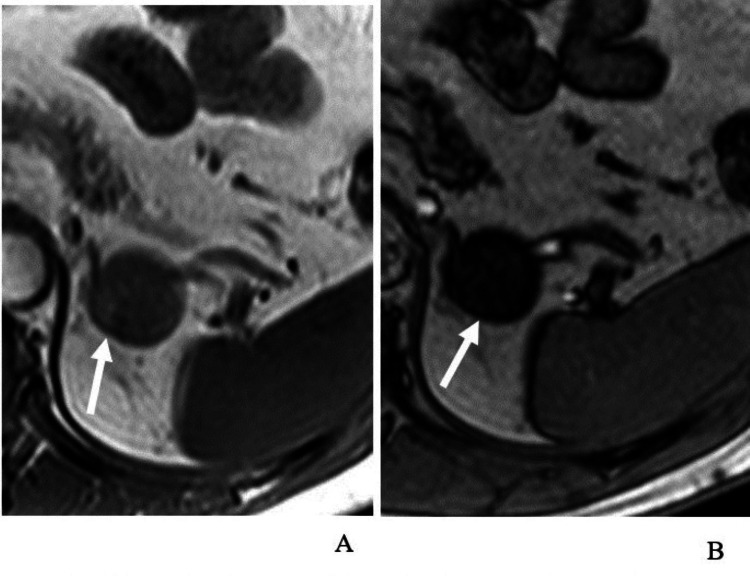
Axial 2D gradient-echo T1-weighted MRI for a 46-year-old man with an incidentally detected adrenal adenoma In-phase (A) and opposed-phase (B) images show diffuse homogeneous subjective signal loss within the adenoma, with a signal intensity index of 39% (white arrows). MRI: magnetic resonance imaging
